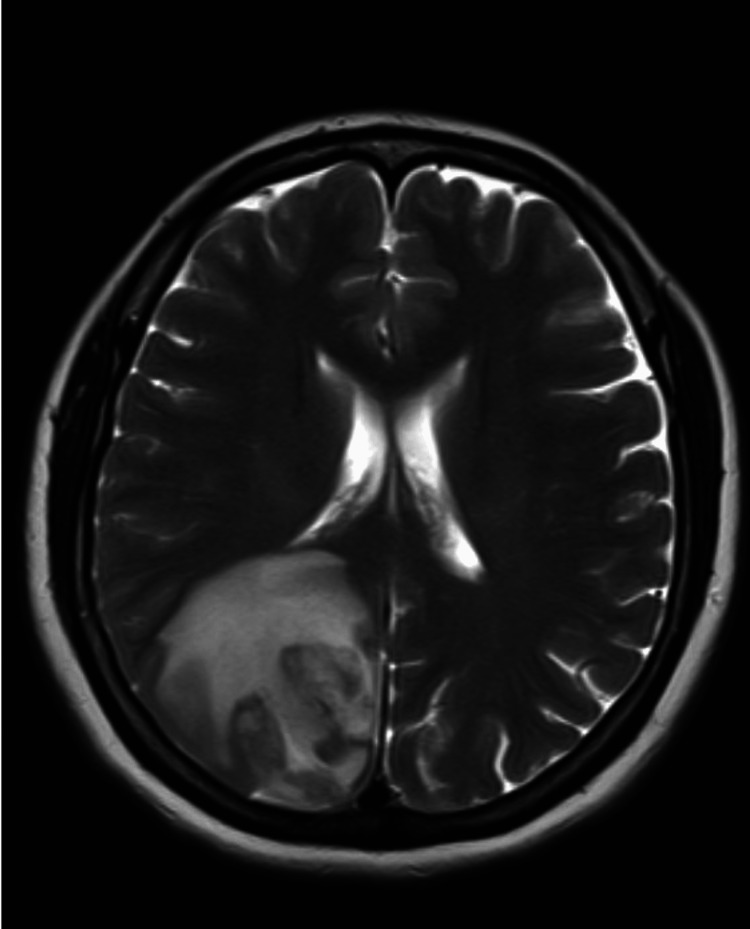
T2-weighted image showing right medial occipitoparietal cortical enhancing lesion with significant adjacent surrounding subcortical/white matter edema.
